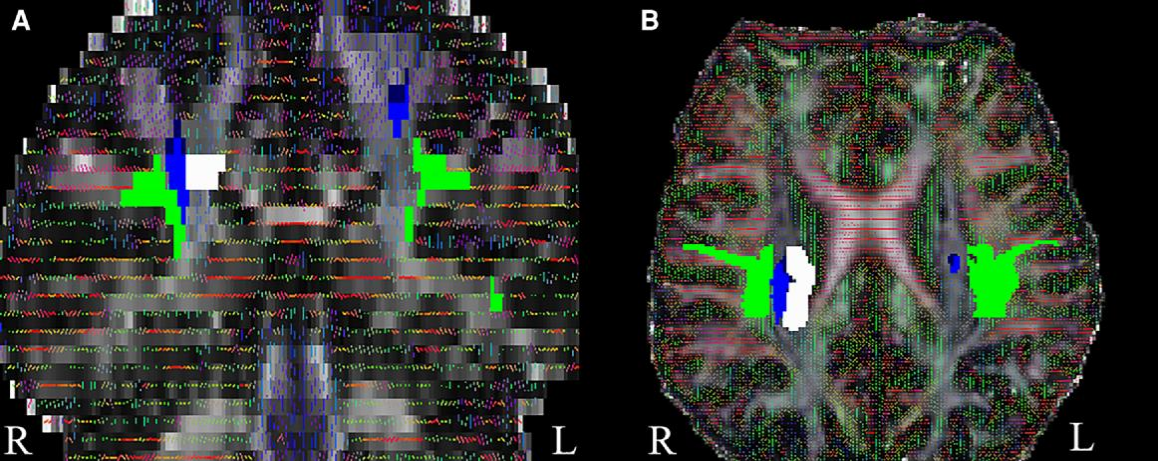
Course deviation of the CST in ALS. Coronal (A) and axial (B) MRI views show deviated location of tractography-identified right corticospinal tract (CST, blue) adjacent to the superior longitudinal fasciculus (green). This is in contrast to the expected (normal) location of the CST (white) averaged from several neurologic controls.
